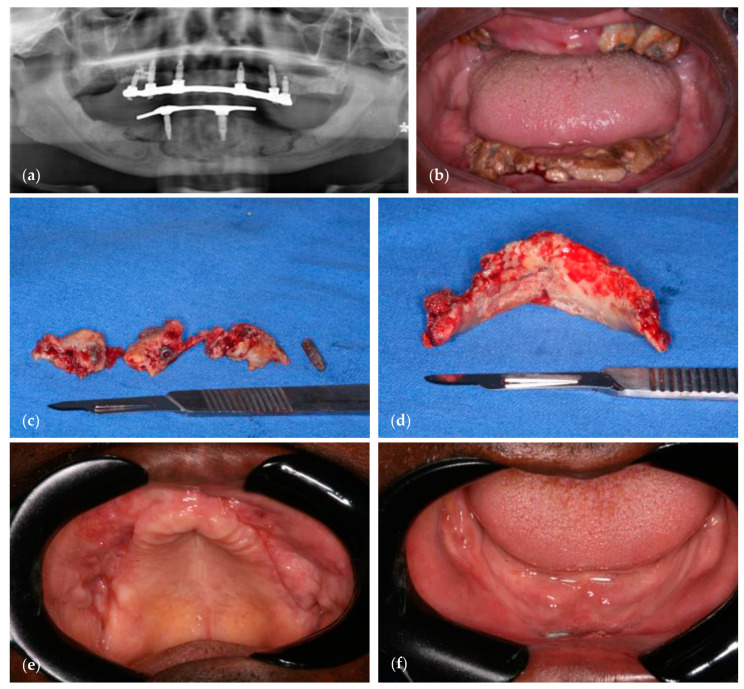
A 59-year-old male patient with multiple myeloma and a history of zoledronate treatment for 3 years, followed by Denosumab treatment for 3 years. Treatment stopped a year before the patient had multiple implants in the maxilla, and then in the mandible 4 months later, for a full denture. Shortly after, massive osteonecrosis occurred in both the mandible and maxilla. (a) Panoramic reconstruction of cone-beam computerized tomography of the mandible and maxilla, demonstrating sequestration of alveolar bone in both jaws; (b) intraoral photograph showing exposed necrotic bone; (c,d) sequestered bone removed from the maxilla and mandible, respectively; (e,f) intraoral photographs one month after surgical excision and primary closure of the maxilla and mandible, respectively, showing complete mucosal healing.
